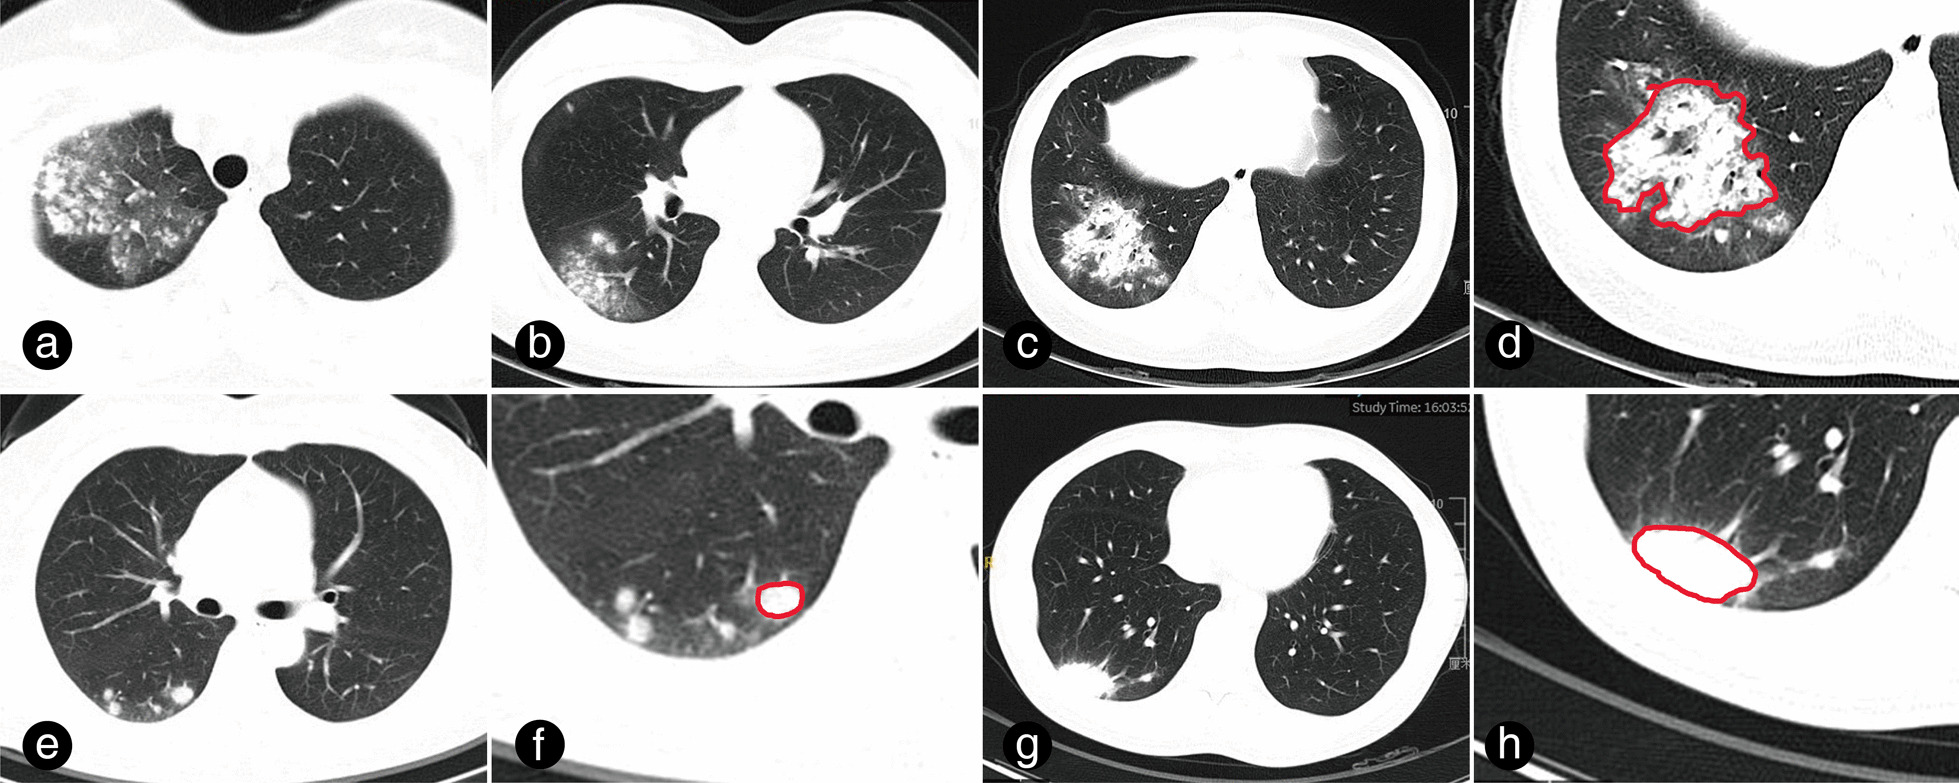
Measurement of the pulmonary lesion area and radiodensity. a–d The case of a 22-year-old female patient diagnosed with cryptococcal granuloma through endobronchial ultrasound-guided trans-bronchial needle aspiration (EBUS-TBNA) is presented. The patient exhibited multiple nodular and patchy lesions in the right lung a–c. The largest lesion was delineated with a red line in panel d, where its radiodensity (-173 HU) and area (14.12 cm^2^) were measured. e, f A 50-year-old female patient with multiple nodules in the right lower lobe and a serum CrAg titre of 1:5 was examined. The largest lesion was identified and its radiodensity (− 165 HU) and area (0.79 cm^2^) were measured using the same method as before. g, h A 44-year-old male patient with a serum CrAg titre of 1:2 and a mass lesion in the right lower lobe is described. The largest cross-sectional area of the lesion was marked by red line in panel h, where its radiodensity (− 13 HU) and area (5.06 cm^2^) were measured
